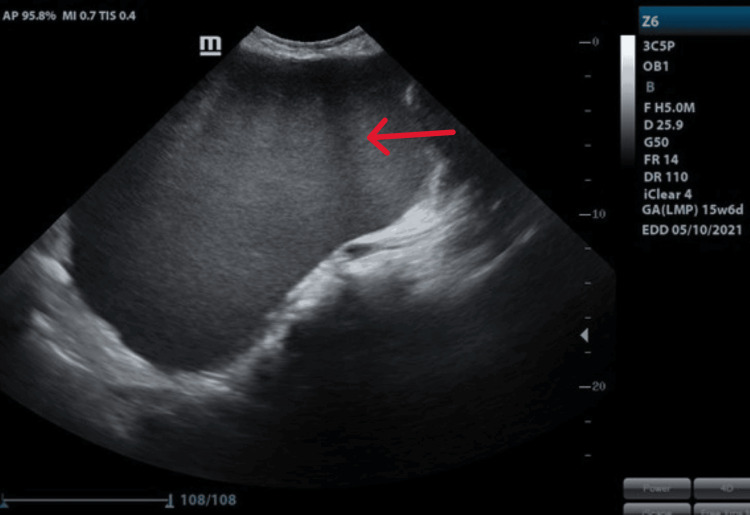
Ultrasound finding of ovarian endometrioma
